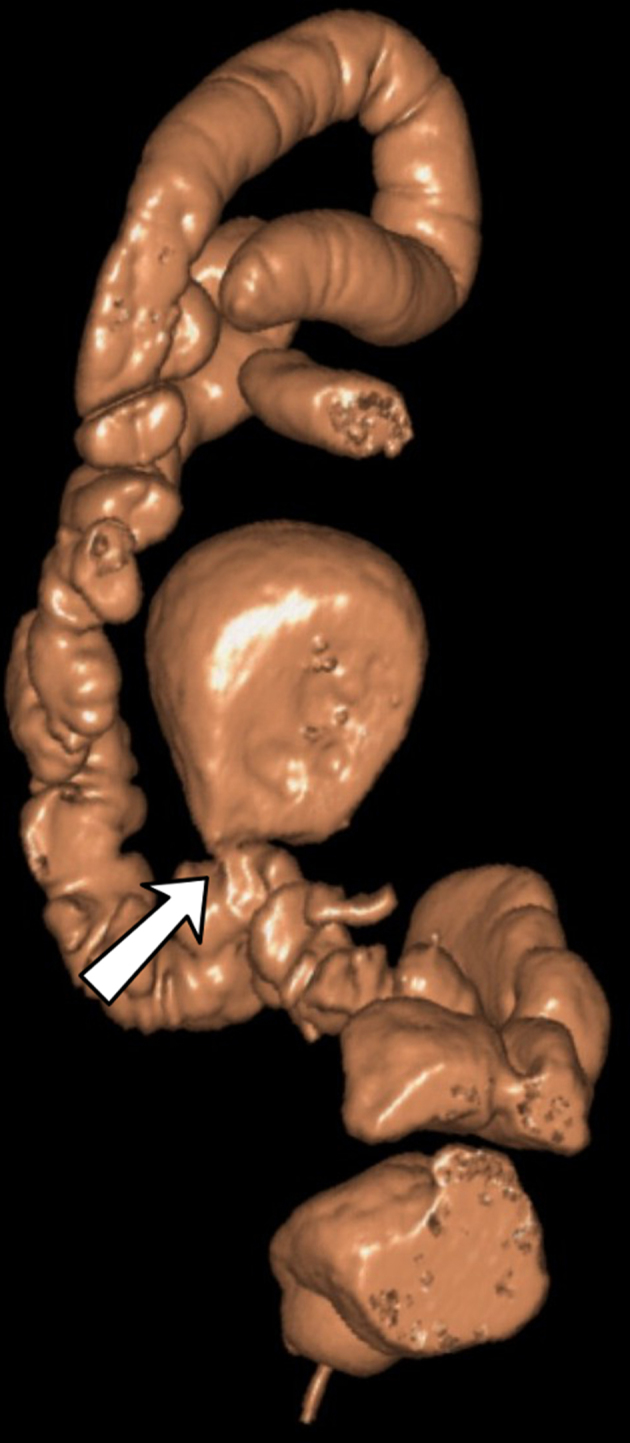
CT 3D colon map, supine position, showing a thin connection of giant colonic diverticulum to sigmoid colon lumen (arrow).
